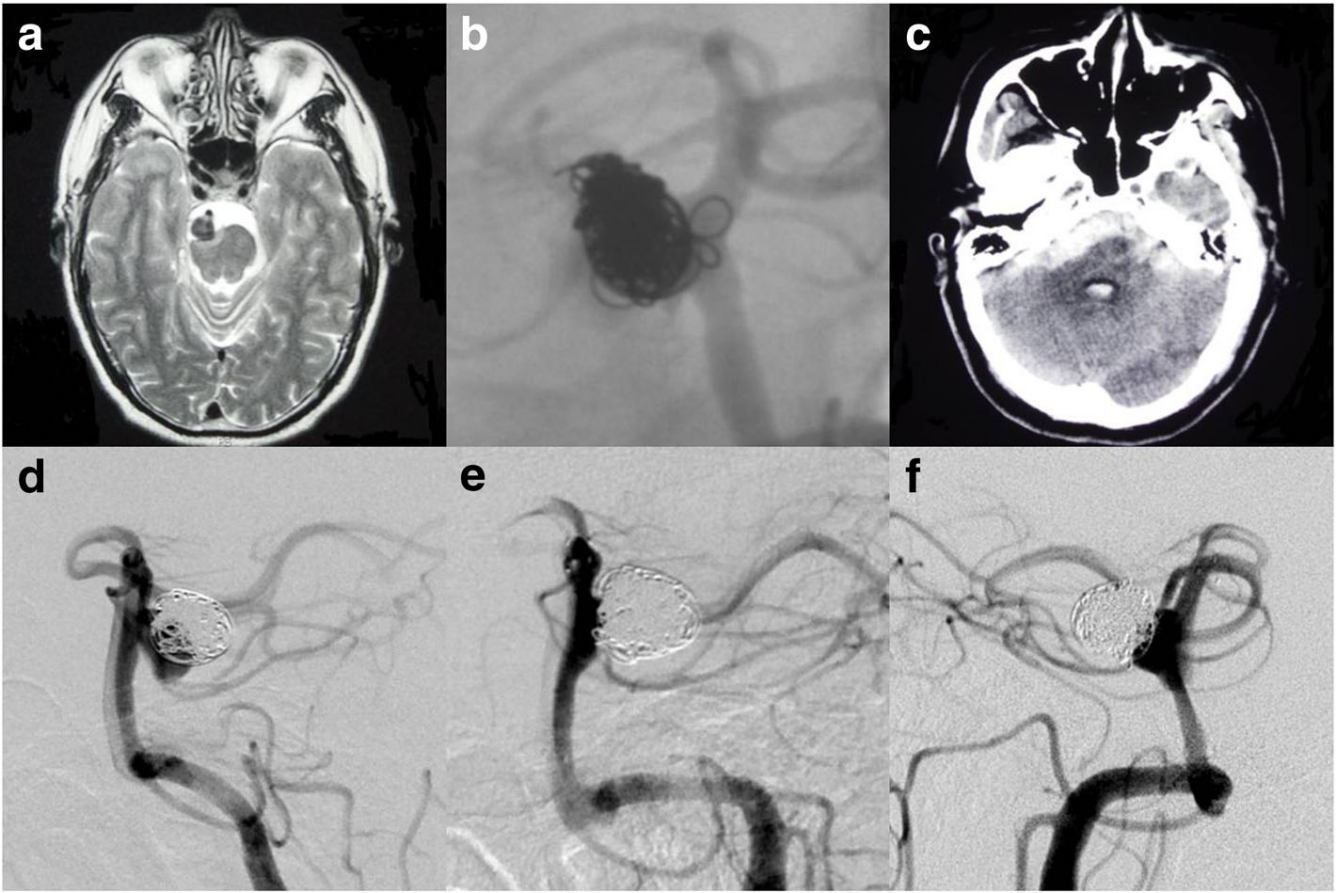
a The MRI indicated an aneurysm in front of the middle segment of the basilar artery. b Control DSA indicated near complete occlusion of the aneurysm. Ten years later, the patient presented with SAH suddenly (c) and DSA confirmed recurrence of the aneurysm (d). e The aneurysm was then treated again with robust packing of coils and an Enterprise stent. f Despite all these efforts, the coils were compacted and inflow zone was again patent as revealed by DSA
